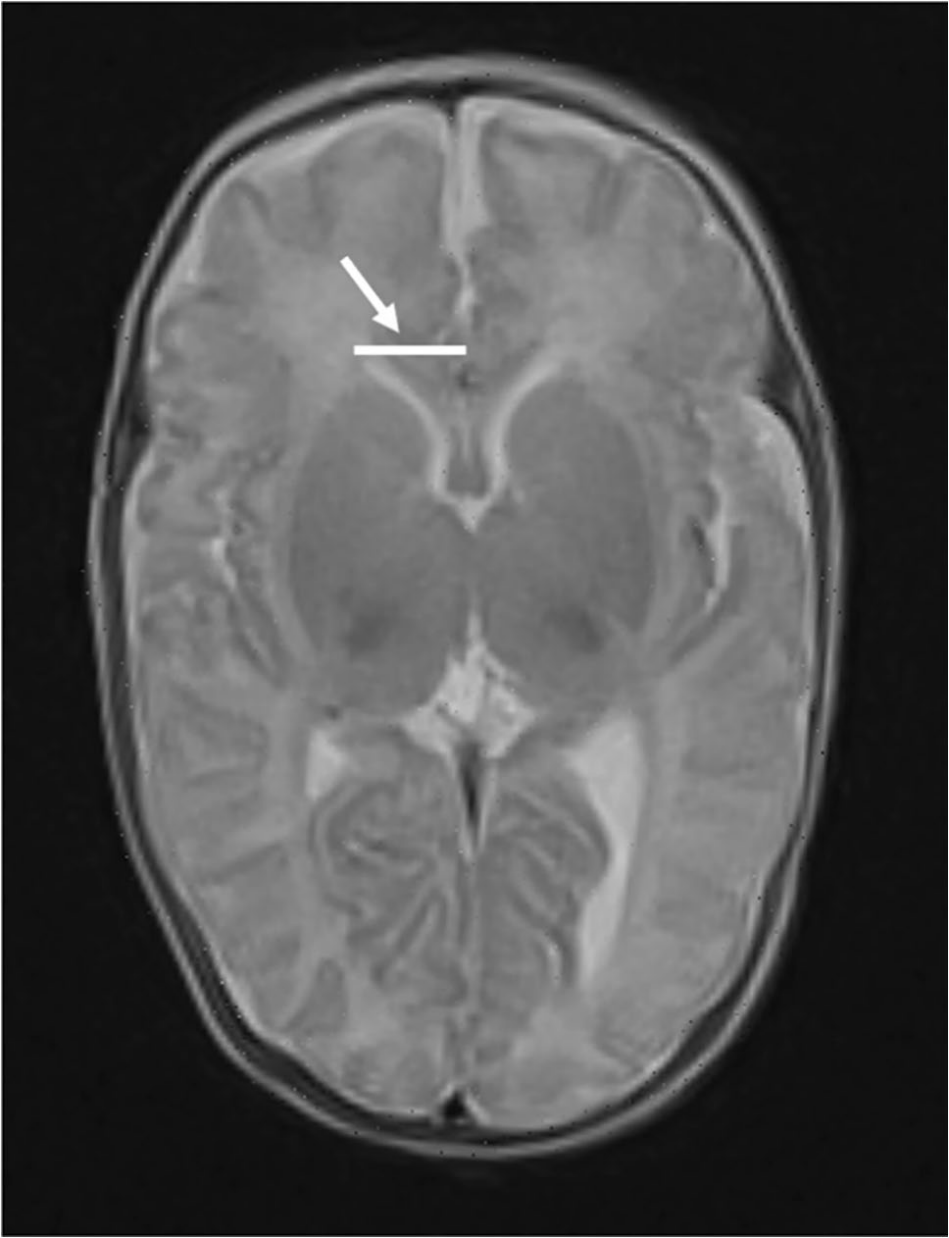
A 12-week-old girl born extremely premature at 28 gestational weeks with magnetic resonance imaging performed at term-equivalent age. Axial T2-weighted image without contrast at the level of the foramen of Monro shows frontal horn width on the right side measured in the axial plane (*arrow*). The infant had no intracranial haemorrhage or other pathology identified on cranial ultrasound in the neonatal period
